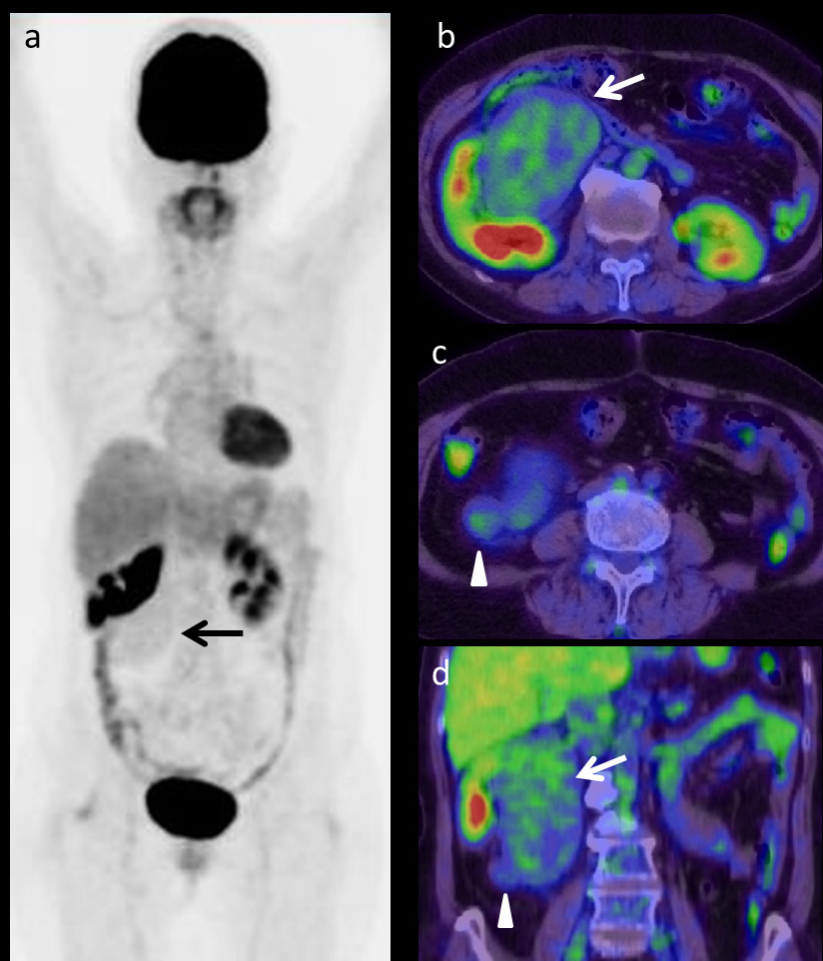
FDG-PET/CT showed low FDG accumulation in the renal tumor on maximal intensity projection (a) and axial (b, c) and coronal (d) PET/CT images. The SUV_max_ values of the main part and the nodular component of the caudal portion of the tumor were 2.3 (b, d, arrows) and 1.91 (c, d, arrowheads), respectively
